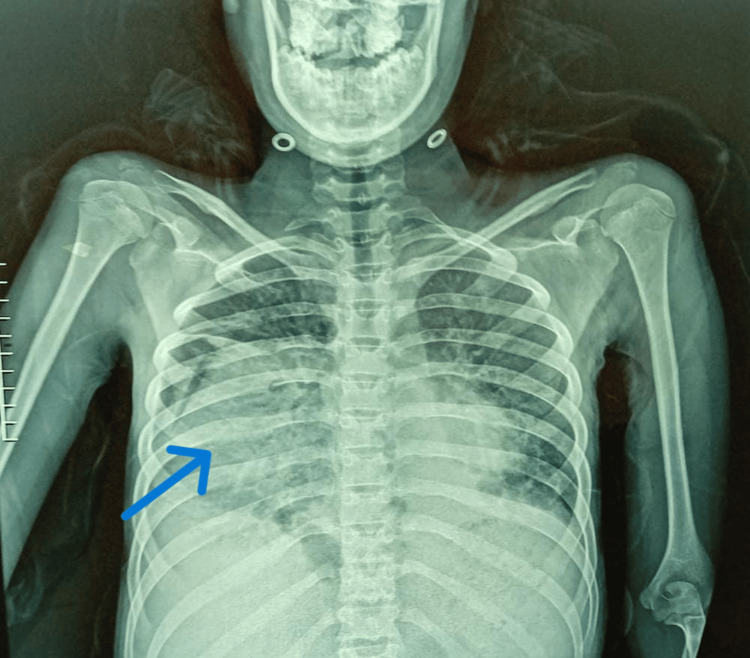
Frontal chest X-ray demonstrating a right lower lobe opacity traversed by air bronchograms (arrow)
